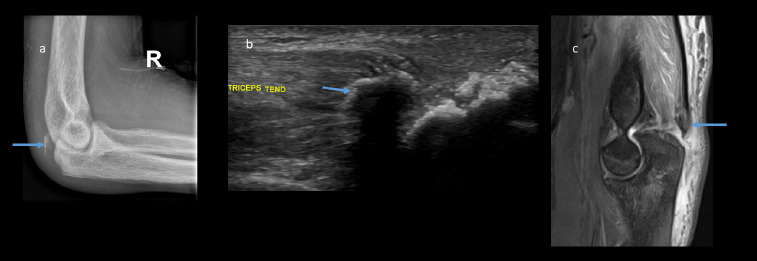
Right elbow a) lateral plain radiograph b) Longitudinal ultrasound c) Sagittal PD FAT SAT MRI showing avulsion fracture of olecranon (blue arrow) from triceps attachment site with associated posterior soft tissue swelling. The right sided avulsion fracture is less displaced compared to the right.
